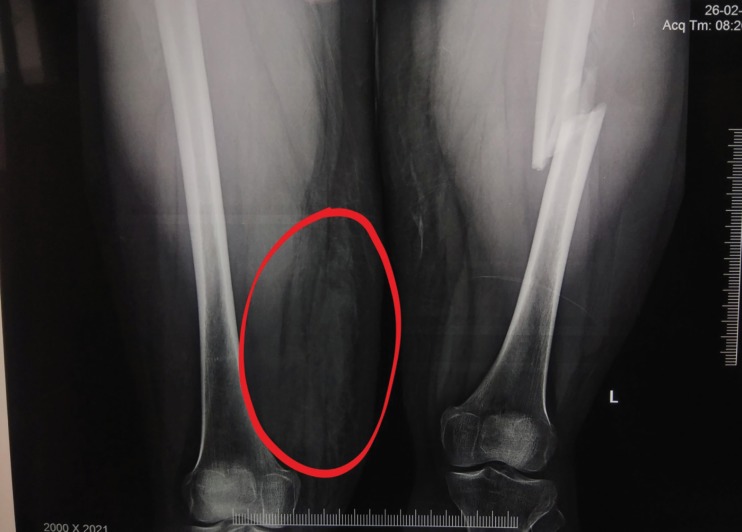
Radiographs showing left femoral shaft fracture and abnormal soft tissue shadow in the medial aspect of the right distal thigh (encircled)
